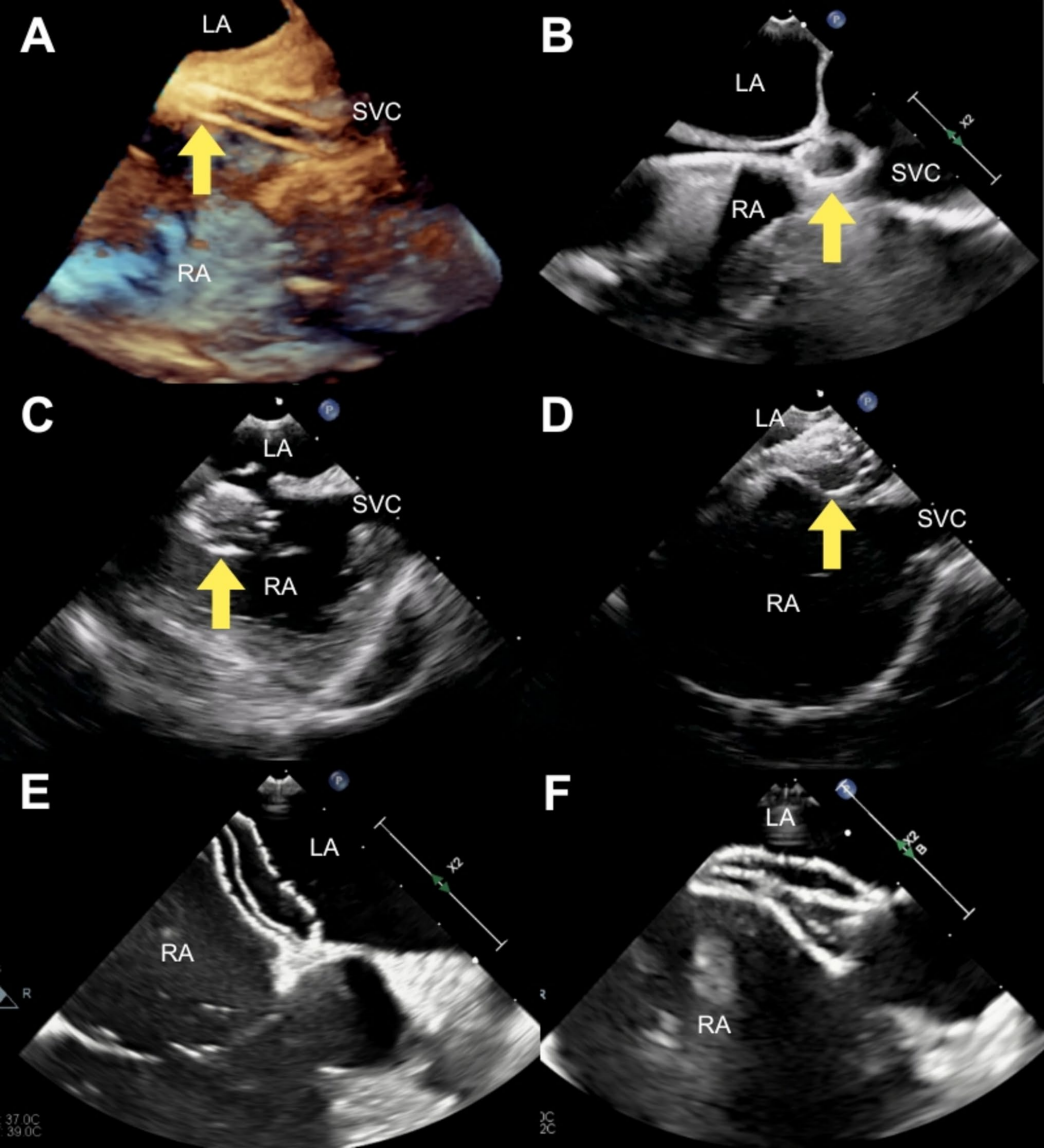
The modified procedure. (A) A 3D transoesophageal echocardiogram image shows the ASD occluder (arrow) advanced within the delivery sheath in the SVC. (B) A small portion of the left atrial disc of the occluder (arrow) is extruded from the end of the sheath in the SVC to form an ‘onion’. (C) The ‘onion’ and delivery sheath assembly (arrow) is rotated towards the ASD. (D) The ‘onion’ and delivery sheath assembly (arrow) is manoeuvred across the defect into the left atrium. (E) The left atrial disc is deployed and positioned. (F) The right atrial disc is deployed in the usual way. Key: SVC: superior vena cava; RA: right atrium, LA: left atrium
